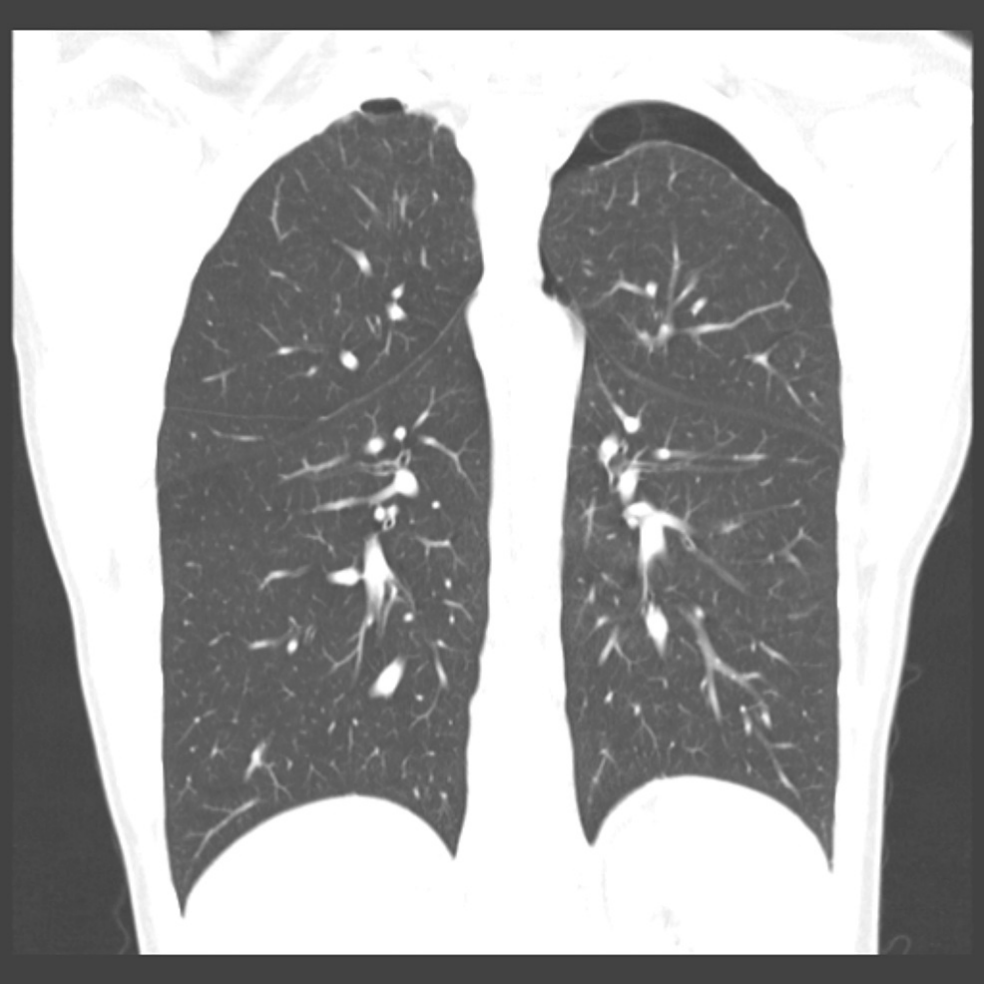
Patient's high-resolution chest computed tomography showing bilateral minor apical bullae and a small left-sided pneumothorax.
